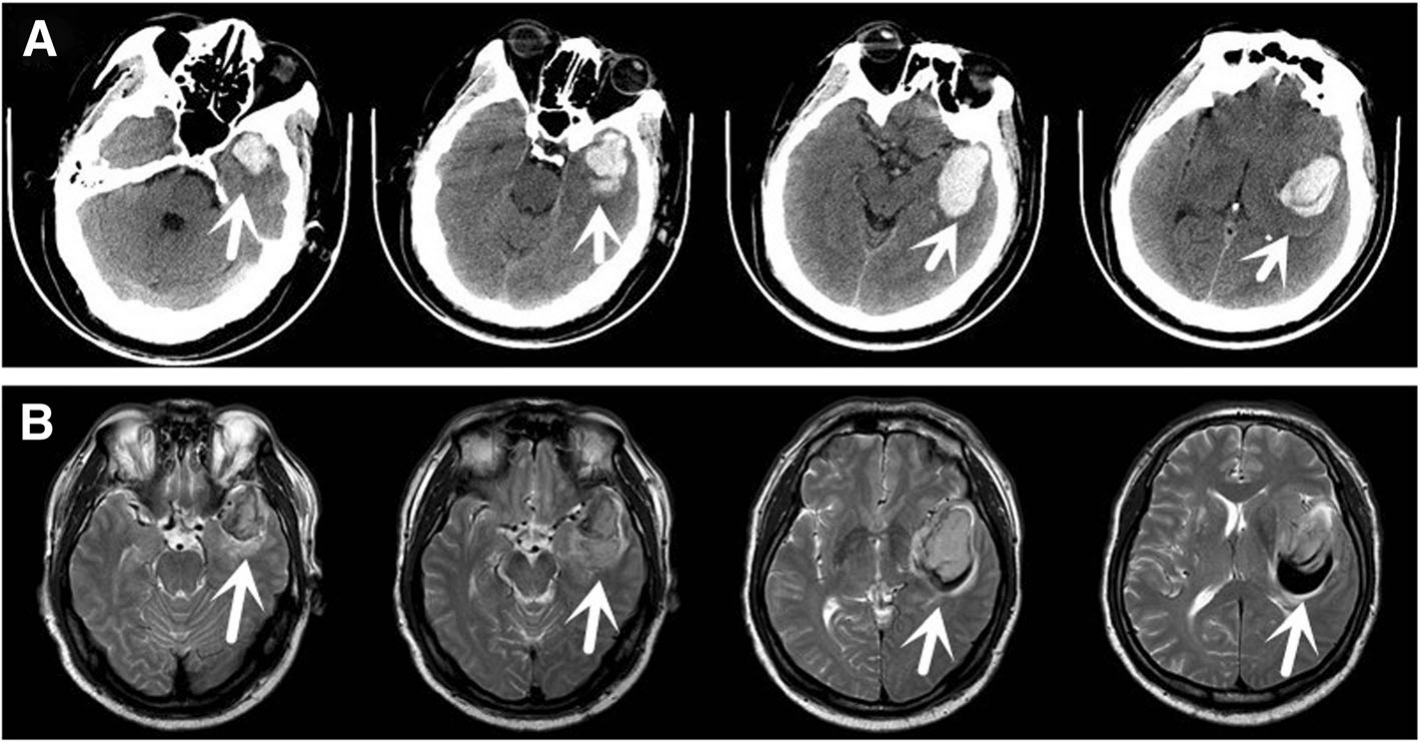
a Clump-like high-density shadows were visible in the left temporal lobe, and fissures of the left lateral ventricle and the left lateral fissure were narrowed due to compression. b Head MRI taken on the next day after onset showed that there was no significant vascular malformation
